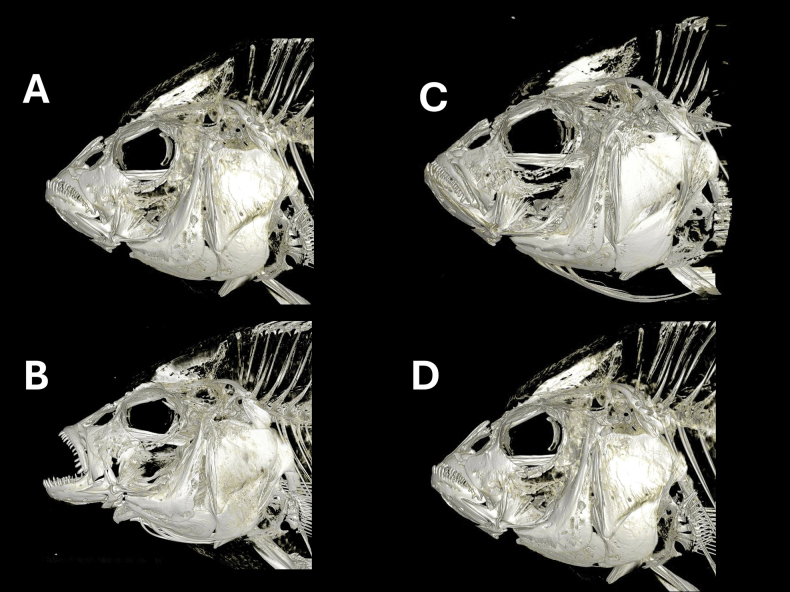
CT scans of the skull of *Labrochromismawe* sp. nov. and *Labrochromismawepili* sp. nov. All are males from top left A*L.mawe* sp. nov., NMBE 1111873, Python Island B*L.mawe* sp. nov., NMBE 1111871, Python Island C*L.mawe* sp. nov., NMBE 1111893, Kissenda Island D*L.mawepili* sp. nov., NMBE 1111906, Makobe Island, Lake Victoria, Tanzania.
